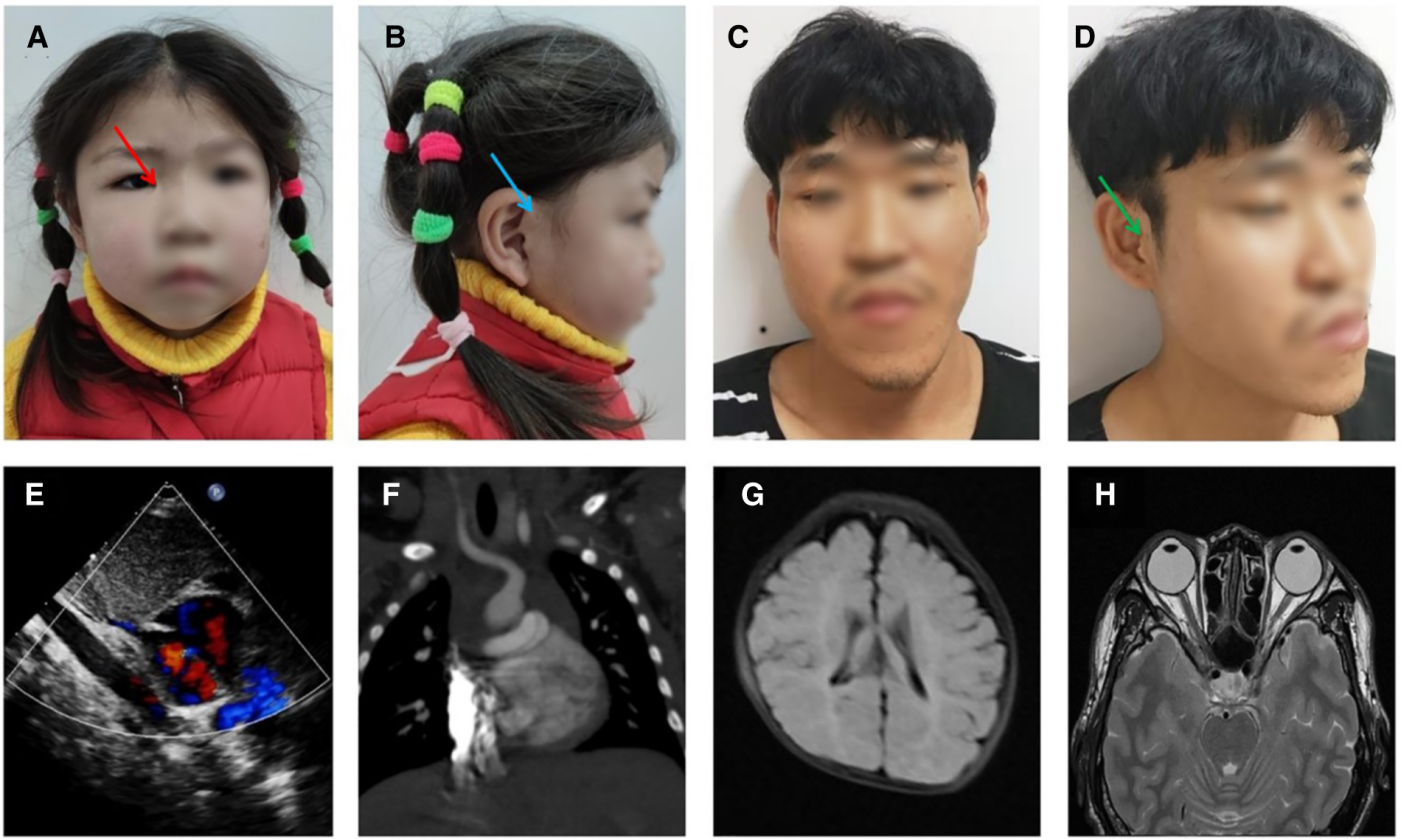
Craniofacial features and imaging results of the proband and father. (A,B) photograph of the proband at 4 years and 5 months: facial asymmetry, epicanthus (red arrow), and preauricular pits (blue arrow), (C,D) photograph of the father: facial asymmetry, epicanthus, and preauricular pits and kin tags (green arrow), (E) heart ultrasound of proband (1 M), (F) pulmonary artery CTA of the proband (4 M), (G) head MRI of the proband (4 M), (H) head MRI of the father.
